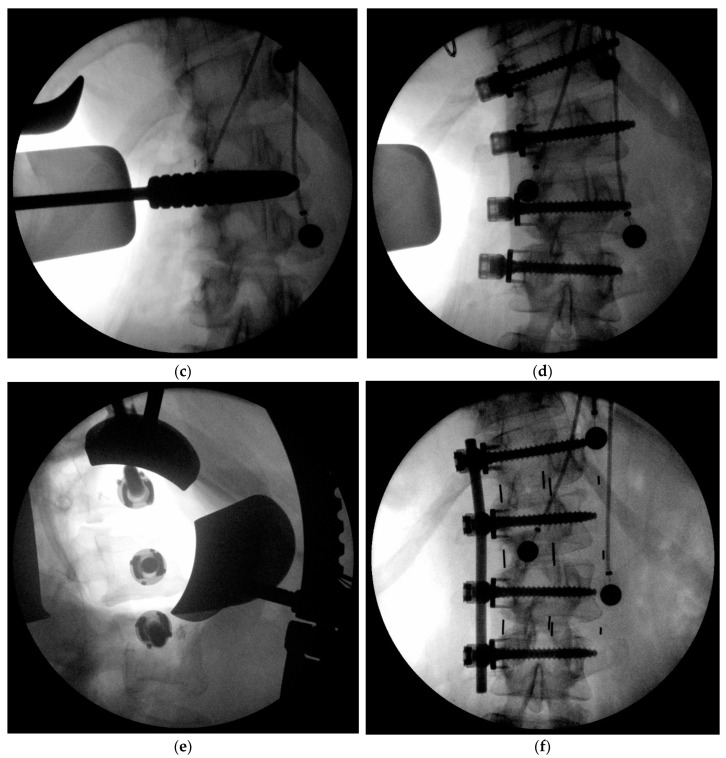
Intraoperative images of the less invasive technique to the concave apex of the curve (a), The arrows indicate the planned trajectories and location for the wound incision. A radiographic marker is placed to confirm levels (b). Coronally tapered trials are used to determine interbody implant sizes starting at the end vertebral levels and working subsequently to the apical levels (c). The screw staple implants are then placed at the end vertebra first and then subsequently placed through the apical vertebral levels (d,e). Distraction is then applied between each segment and the rod secured to the transverse vertebral body screws (f).
